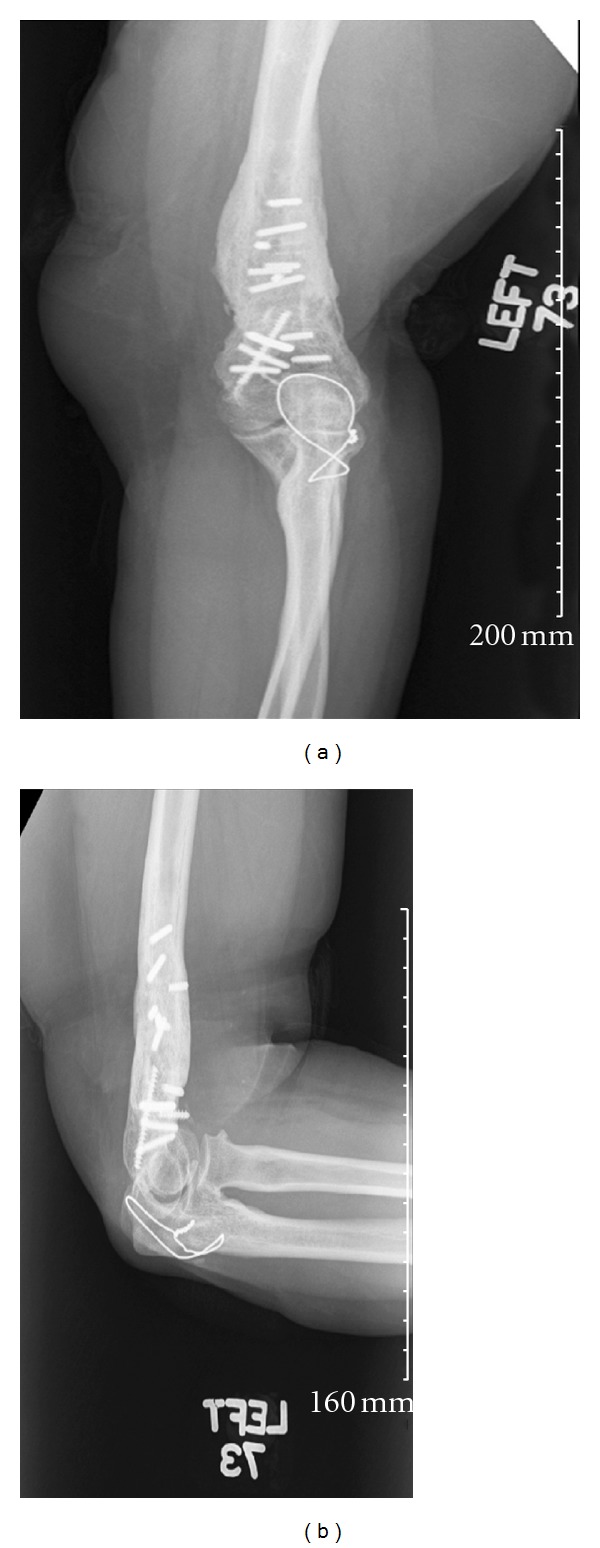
Broken hardware often poses a difficult problem and subsequent removal may increase surgical time and complexity of future surgeries. (a) shows an X-ray of an elbow (AP view) with broken and retained screws. (b) shows the same elbow in the lateral position.
